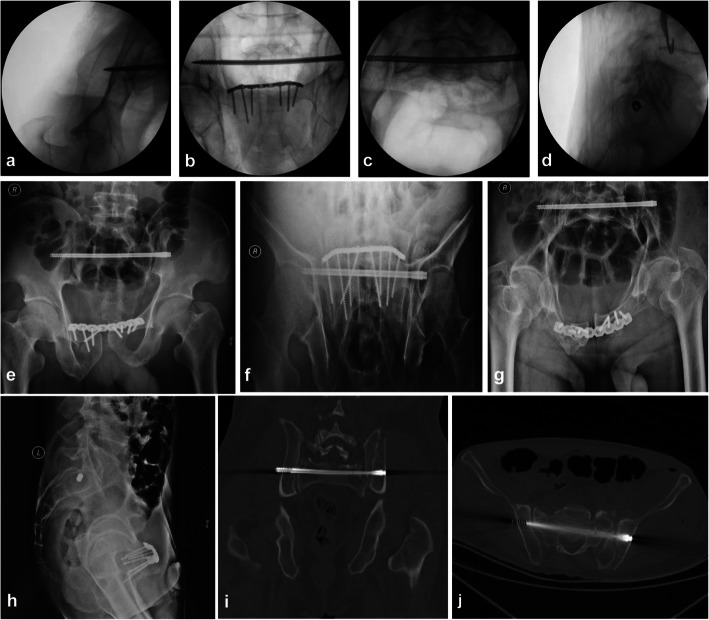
Ensuring the position of the screw by the inlet, outlet and lateral views again, and taking an over-the-top view to assess screw length and far cortex penetration of the iliac (a–d). As shown in the radiographs and CT scans, the position of the transiliac-transsacral screw was satisfied except for the threads protruding through the contralateral ilium (e–j)
